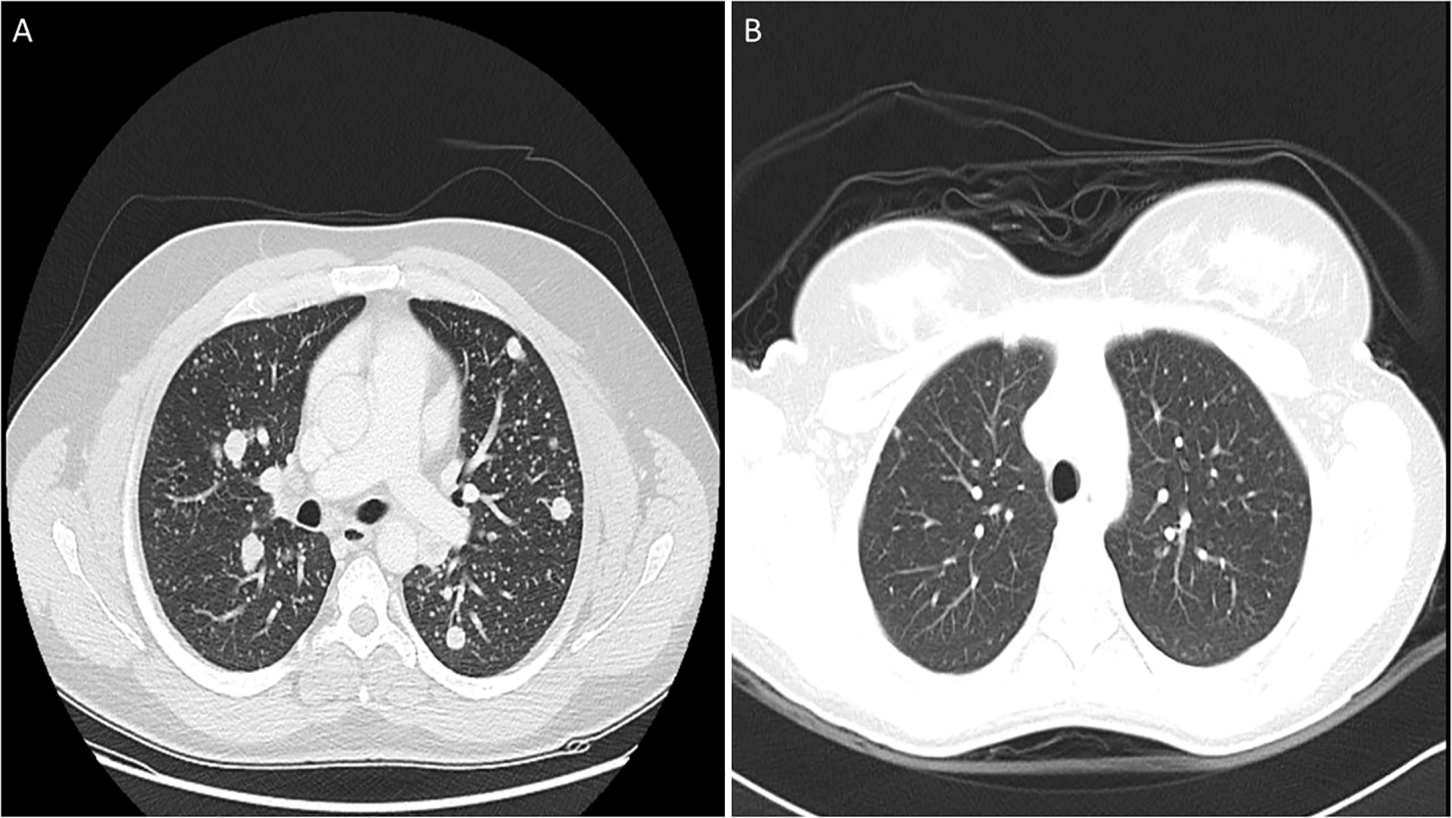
Computed tomography (CT) scan images of lung metastasis in pediatric differentiated thyroid carcinoma patients showing macronodular (A) and micronodular (B) pattern.
